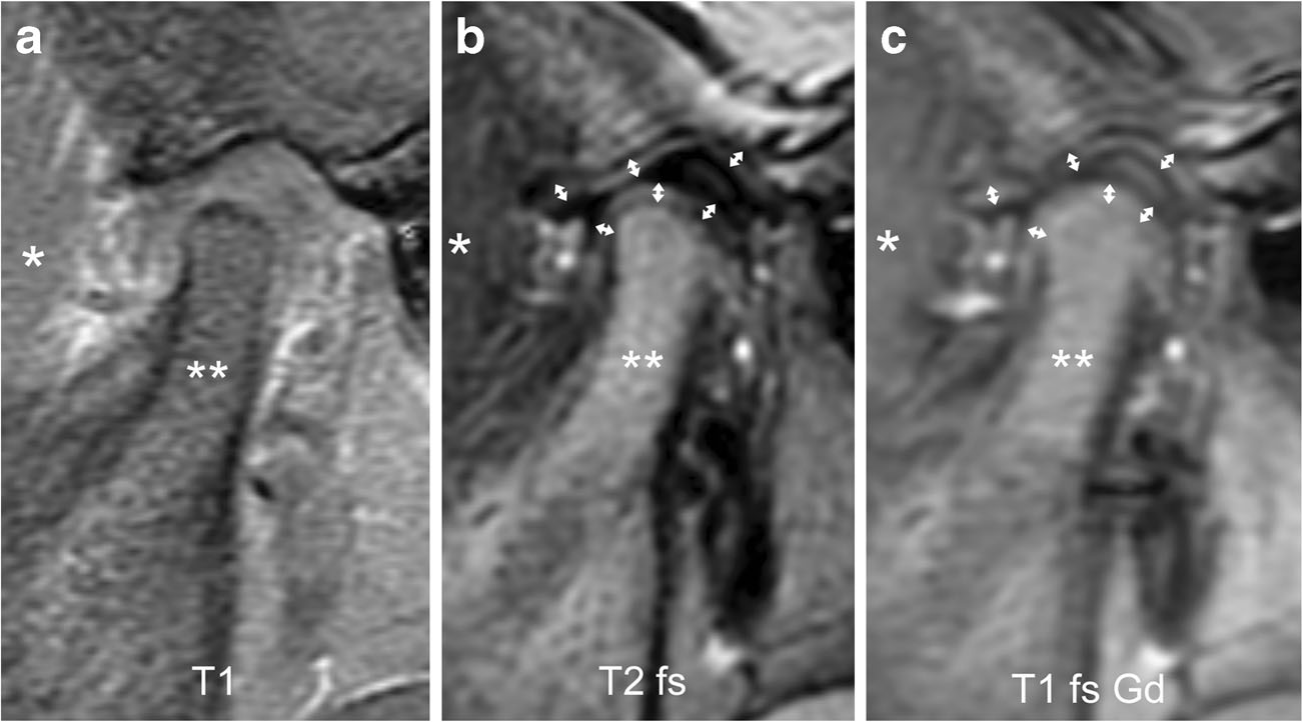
No synovial thickening (grade 0) and no joint enhancement (grade 0) on sagittal oblique images in a 3-year-old boy. a There is a large proportion of haematopoietic marrow in the mandible, which is shown as low signal intensity () similar to that of muscle tissue (*) in an unenhanced T1-weighted gradient echo image without fat saturation (T1, TR/TE 300/4.2 ms, flip angle 80°). b-c The width of both joint compartments (including articular cartilage, synovium and joint space) between the bony articular surface and disk is smaller than 1 mm (small double arrows) on fat-saturated T2-weighted image (TR/TE 5,400/77 ms, b) and on contrast-enhanced fat-saturated T1-weighted image (TR/TE 670/10 ms, c). Postcontrast signal intensity of the joint spaces is similar to that muscle (*). There is higher signal intensity compared to that of muscle (*) in the fluid-sensitive image (b) and postcontrast fat-saturated T1-weighted image (c). T1 T1-weighted, T1 fs Gd contrast-enhanced fat saturated T1-weighted, T2 fs fat saturated T2-weighted
